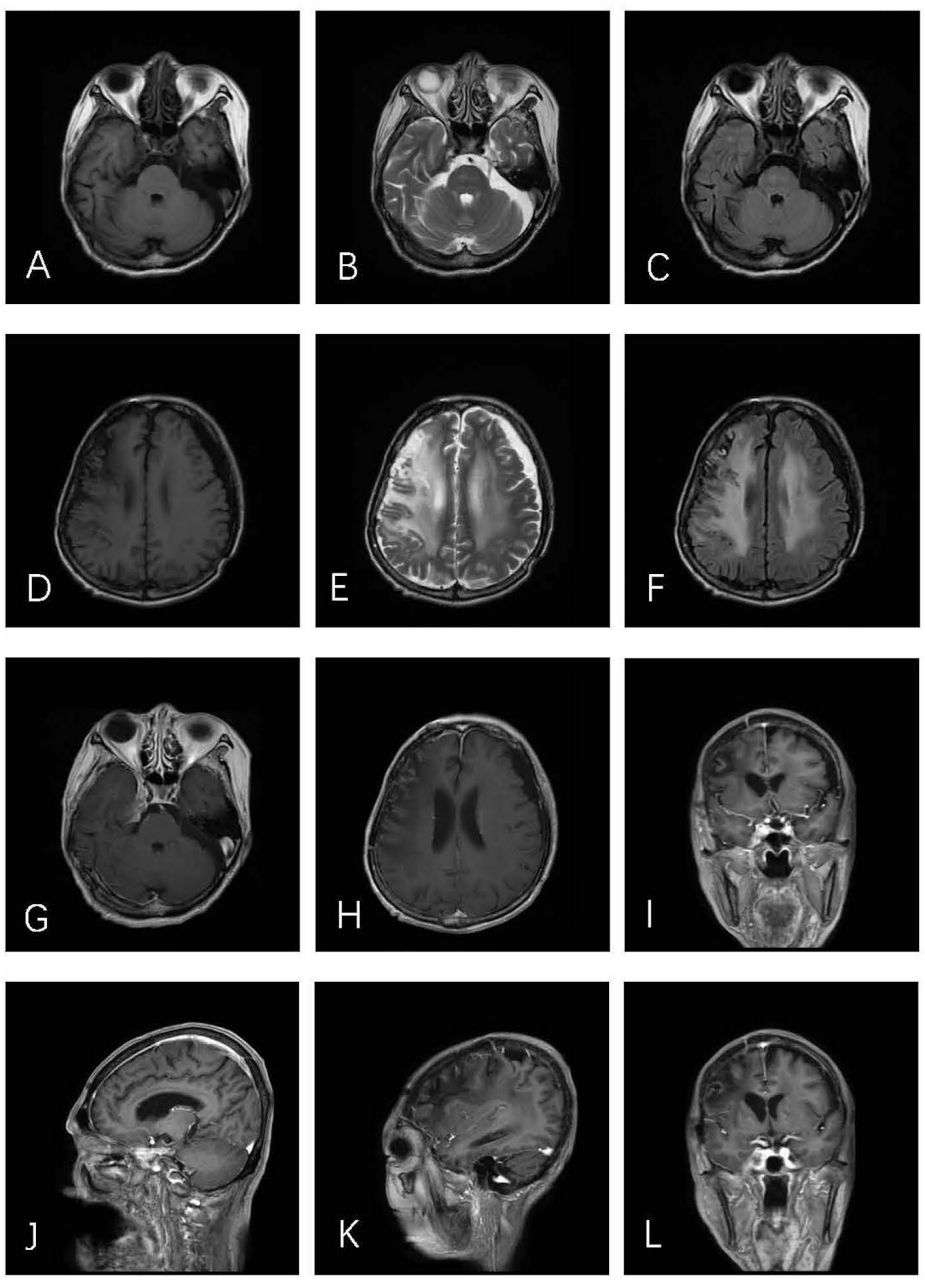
Axial T1WI (A), T2WI (B), and T2–FLAIR (C) demonstrate postoperative alterations in CS. In axial T1WI (D), T2WI (E), and T2–FLAIR (F) images, an obvious edema zone can be seen in the right parietal frontal brain, which may be related to brain changes after radiotherapy. No tumor recurrence was found in post–contrast T1–weighted (G–L) images.
